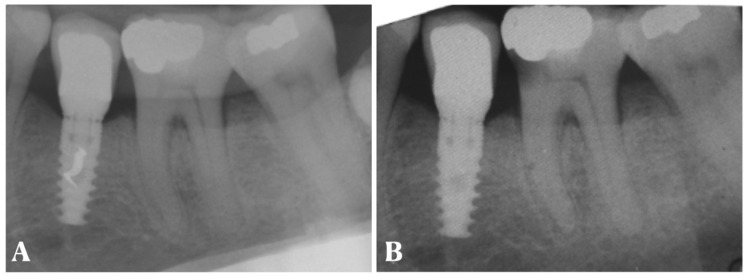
Periapical radiographs: (A) T4 (12-month follow-up); (B) T5 (5-year follow-up). The patient did not attend the control visits. A caries was identified under the old restoration in the mesial portion of the adjacent tooth.
